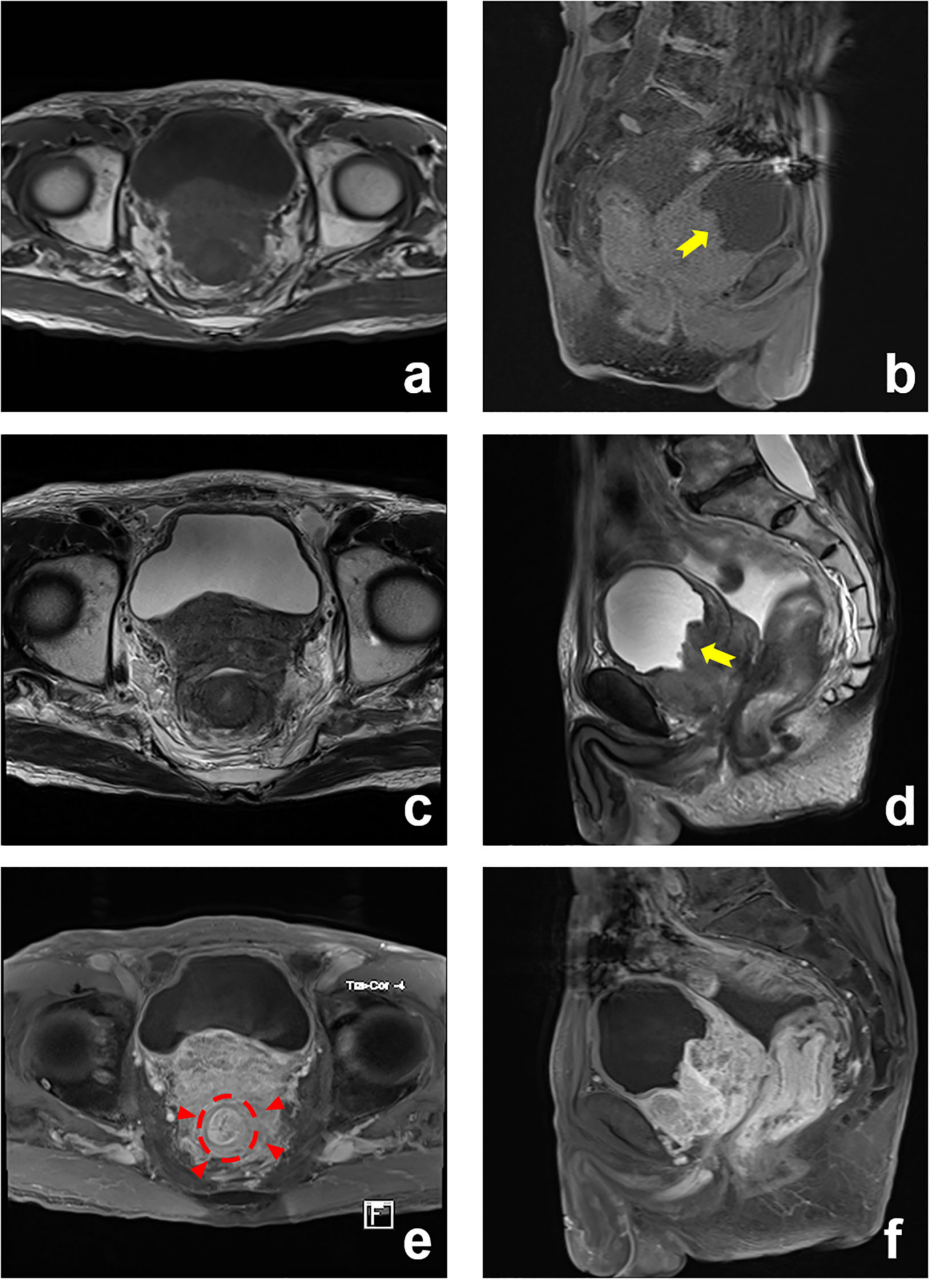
Prostate MRI: enlarged prostate with transition zone enlargement. Posterior extension: encasement of the rectum (“target sign”) (red arrowed). Anterior extension: invasion of the bladder (yellow arrowed). (a) T1-Weighted (T1W), axial; (b) T1-Weighted (T1W), sagittal; (c) T2-Weighted (T2W), axial; (d) T2-Weighted (T2W), sagittal; (e) T1-Weighted (T1W) C+, axial; (f) T1-Weighted (T1W) C+, sagittal.
